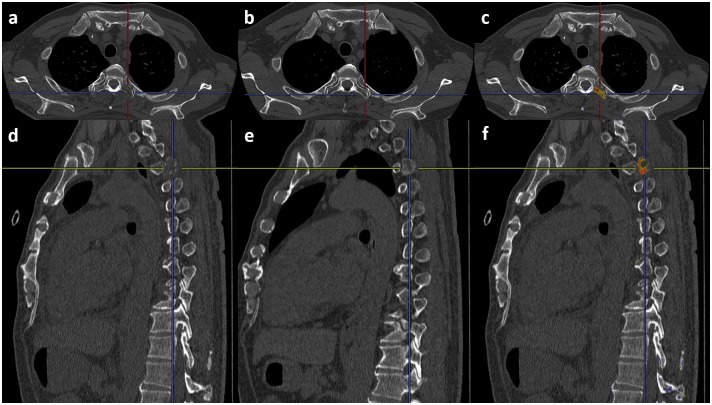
A case of osteolytic metastasis in the left transverse process of the third thoracic vertebra. (a) Follow-up axial image. (b) Initial axial image. (c) Result of the 3D-CT subtraction. The yellow and red colors indicate the metastasis. (d) Follow-up sagittal image. (e) Initial sagittal image. (f) Result of the 3D-CT subtraction. The yellow and red colors indicate the metastasis.
